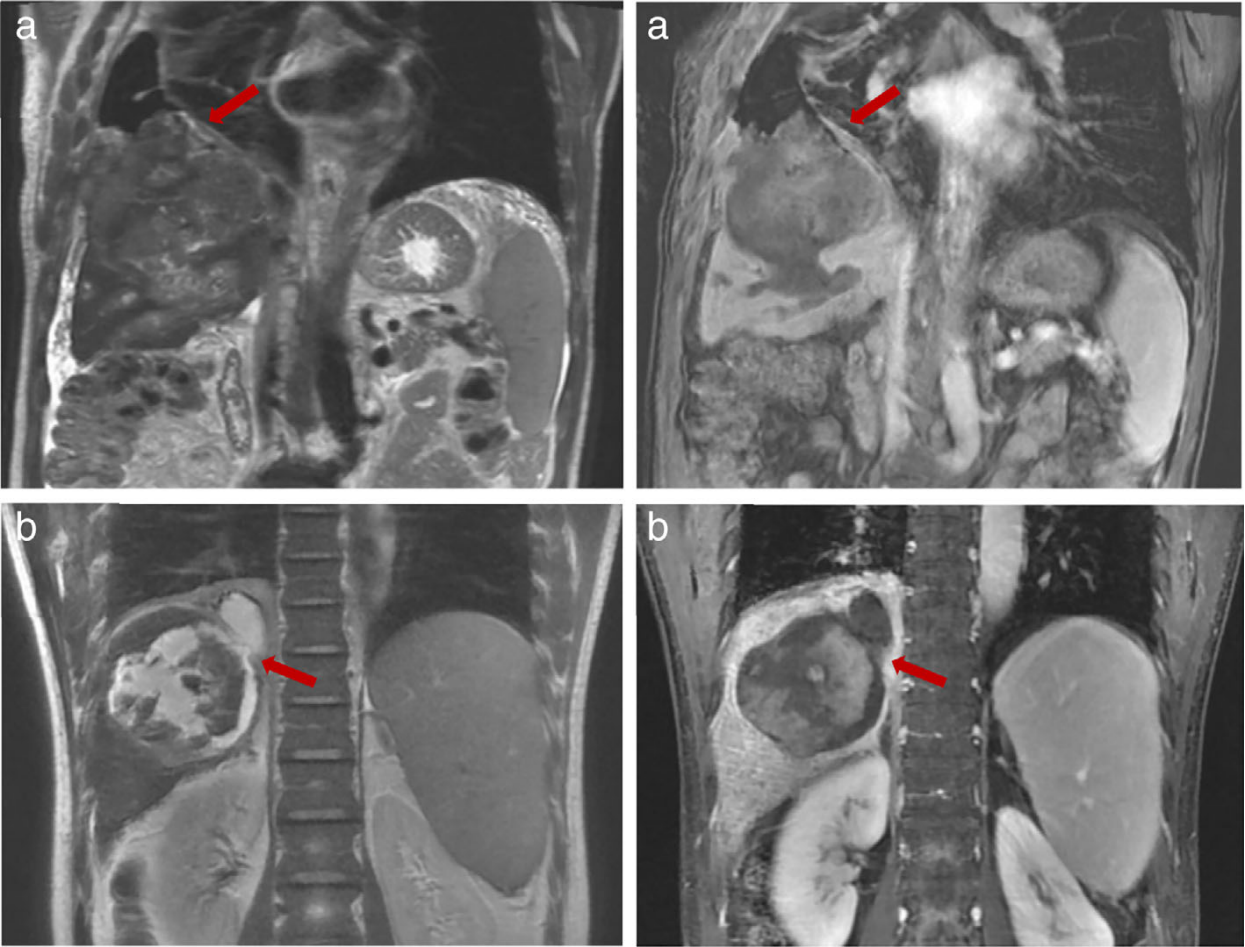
MRI image scans showing the fistulous tract connecting the pleural effusion and biliary lesions in two patients (a, b) with bronchobiliary fistula (red arrows).
